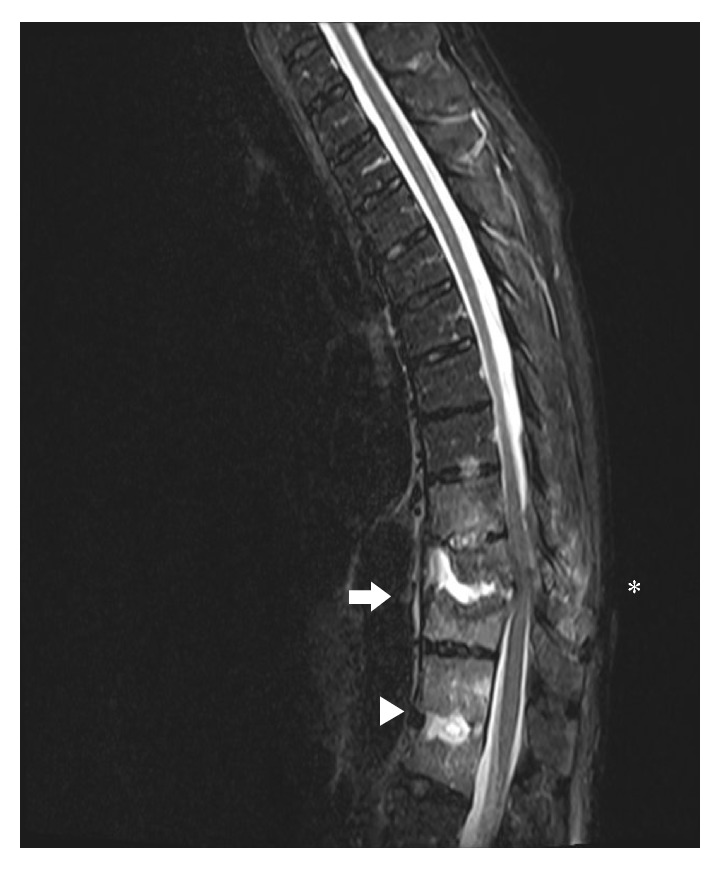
MRI of the thoracic and lumbar spine revealed spondylodiscitis in the thoracolumbar spine, worst at the T10-T11 level (white arrow) where there is associated paravertebral and epidural components with compression of the spinal cord. There is also oedema seen in the spinous processes of T9-T10 and the adjacent paravertebral muscles (Asterisk). Spondylodiscitis was also seen at T12/L1 level (white arrowhead).
